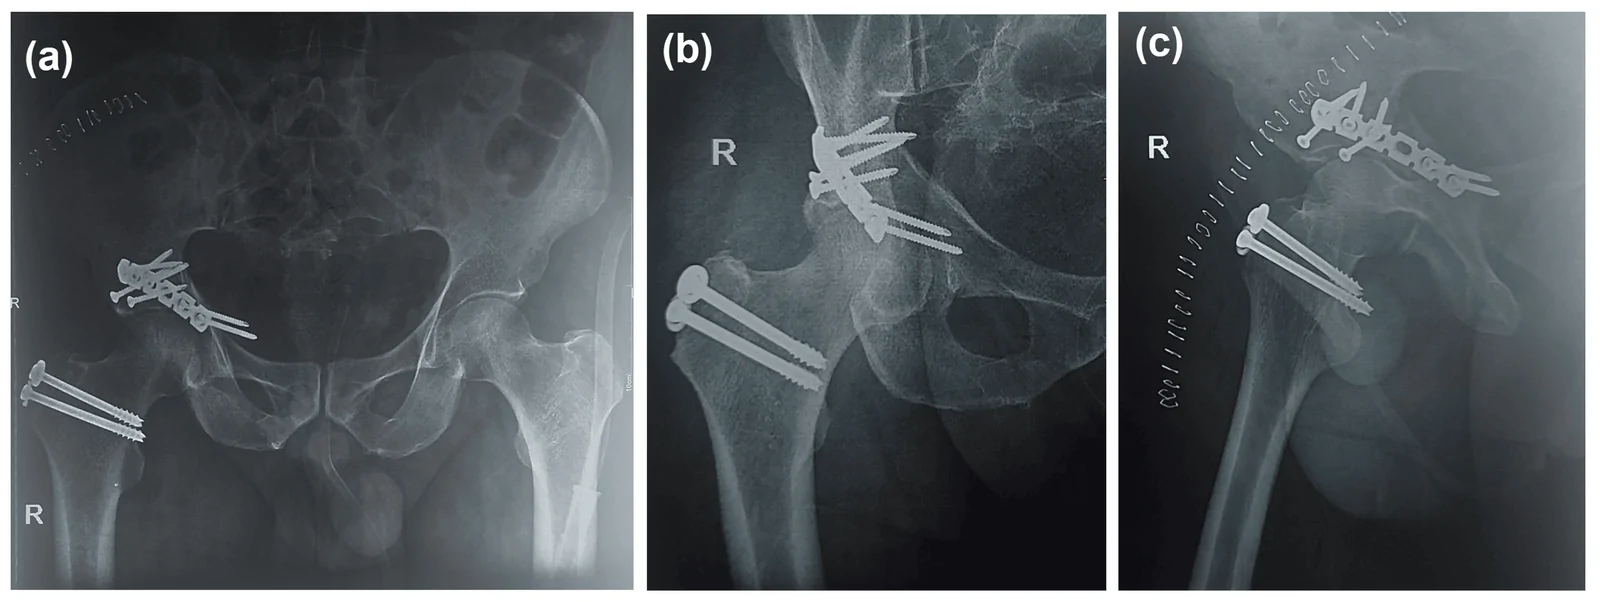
Case 6: Immediate postoperative assessment. (a) Immediate postoperative anteroposterior pelvic radiograph demonstrating anatomical reduction of the associated anterior and posterior column acetabular fracture, with stable reconstruction plate fixation of the posterior column and fixation of the trochanteric flip osteotomy using cancellous screws. (b) Immediate postoperative iliac oblique (Judet) radiograph demonstrating restoration of the posterior column and acetabular roof, with satisfactory reconstruction plate position. (c) Immediate postoperative obturator oblique (Judet) radiograph confirming anatomical reduction of the acetabular columns, restoration of hip congruity, and stable implant fixation.
